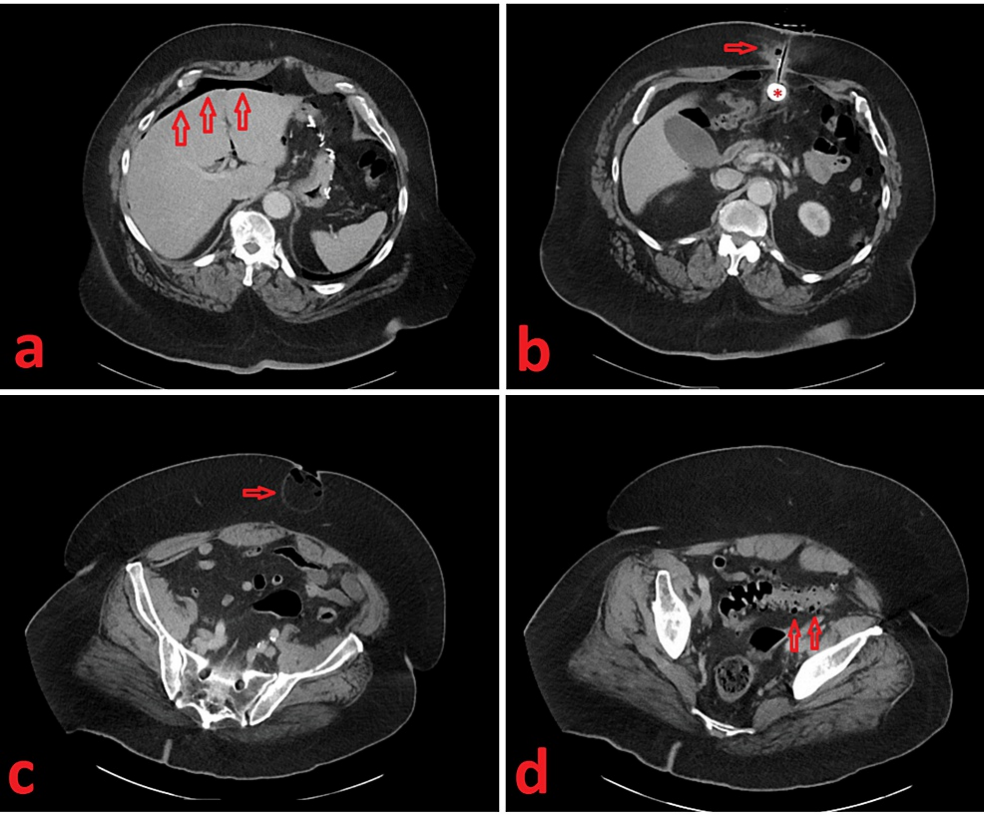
Contrast-enhanced computed tomography (CT) scan of the abdomen and pelvis Axial images of an intravenous contrast-enhanced computed tomography (CT) scan of the abdomen and pelvis demonstrating (a) pneumoperitoneum (arrows), (b) abscess within the subcutaneous tissue along the PEG tract (arrow), and appropriate positioning of the PEG balloon within the gastric sleeve (*), (c) an umbilical hernia containing fat with air and minimal overlying skin with concern for ulceration (arrow), and (d) extensive colonic diverticulosis (arrows).
